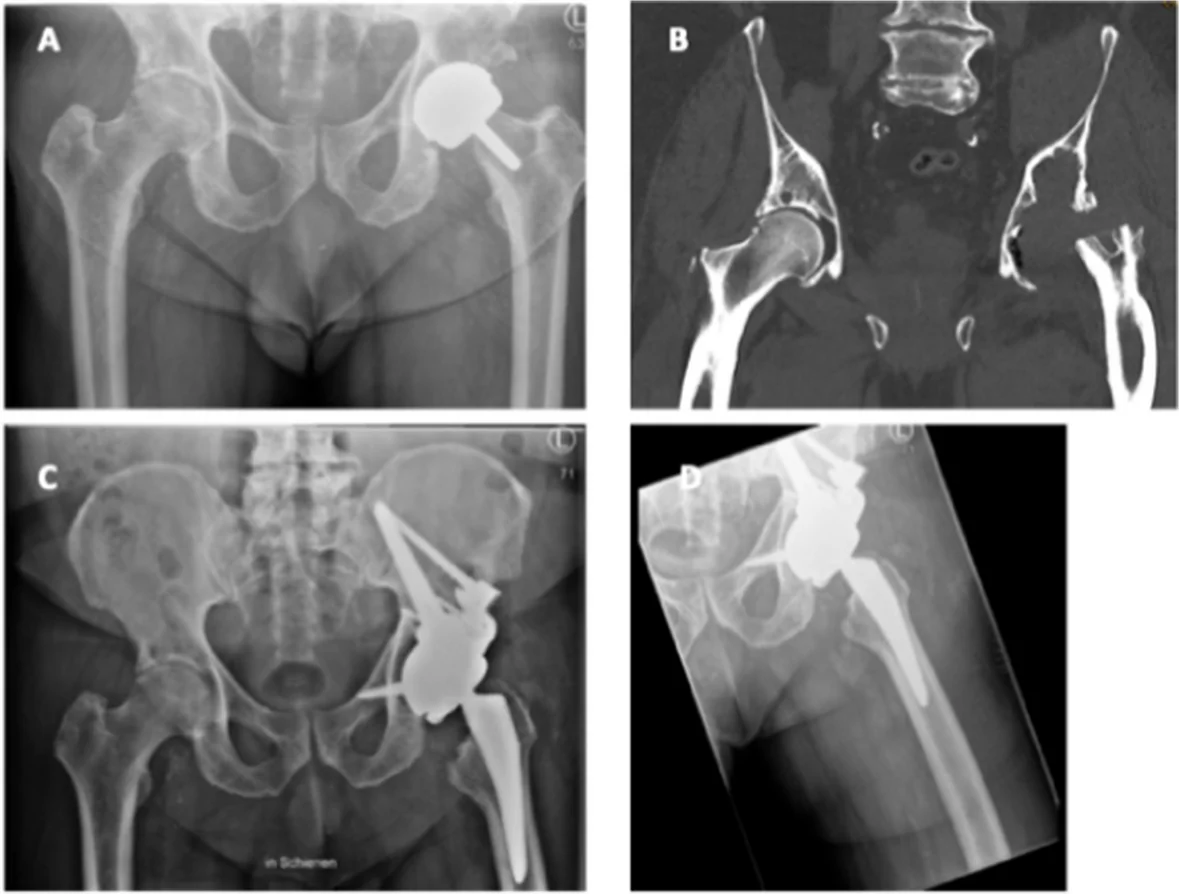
CMAC in extensive cranial osteolysis: imaging follow-up. A Preoperative radiograph demonstrating a loosened McMinn prosthesis on the left side with an associated extensive pseudotumor. B Post-explantation CT imaging showing postoperative conditions with marked metallosis-related granulomatous tissue and extensive destruction of the ilium, particularly involving the acetabular dome, corresponding to ADC type 3C. C Postoperative radiographic evaluation 12 months after implantation of the custom-made acetabular implant, demonstrating stable component positioning and satisfactory osseointegration during follow-up. D Lauenstein view at 12 months showing stable positioning without radiographic signs of loosening
